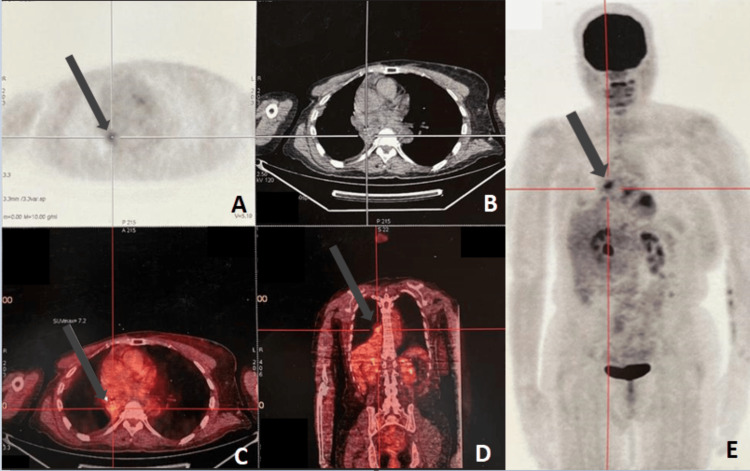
PET/CT scan showing high fluorodeoxyglucose uptake in the right lower lobe bronchus (maximum standard uptake value = 7.2) (A-D) with no other hypermetabolic sites (E).
